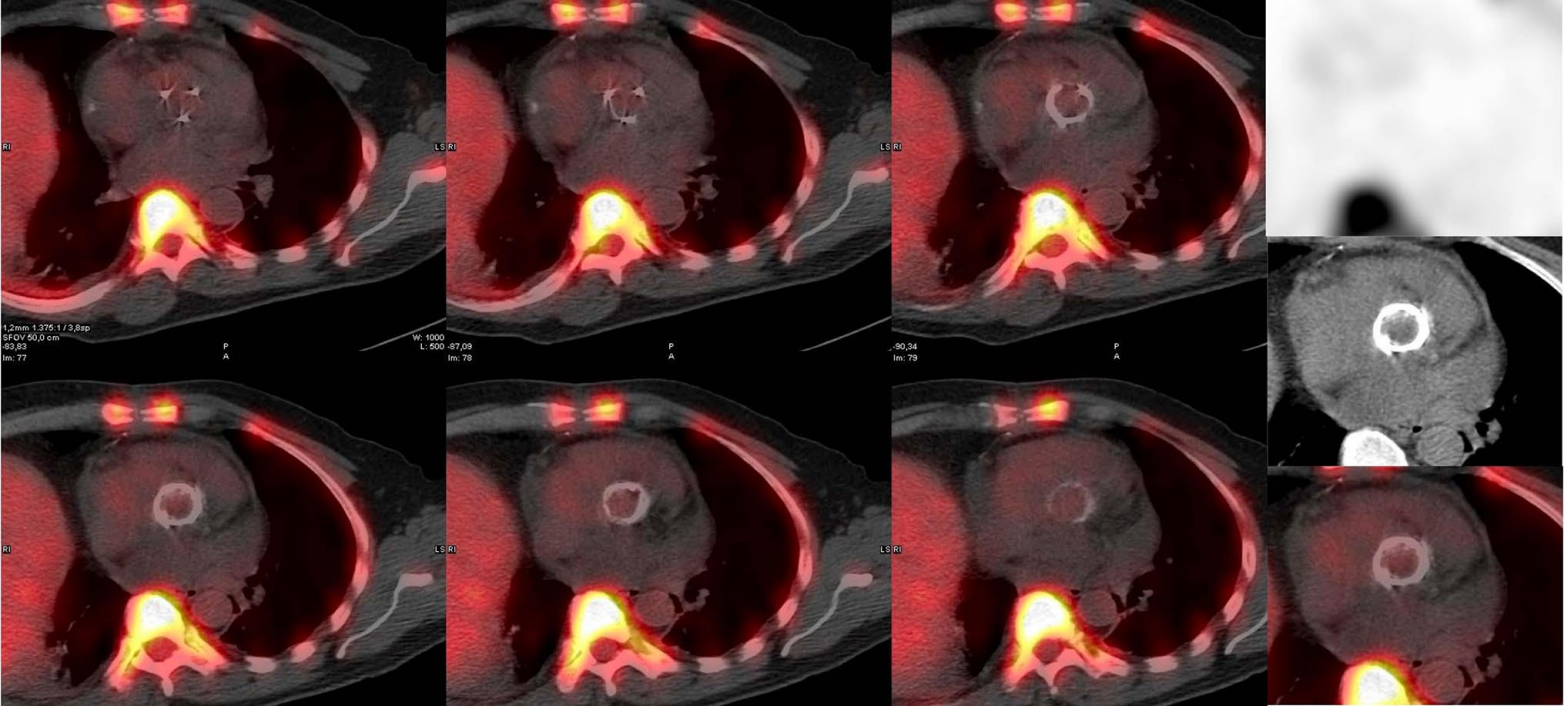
Example of a normal pattern of WBC uptake (^99m^Tc-HMPAO WBC) in a non-infected aortic valve as shown by the re-oriented SPECT/CT images acquired 24 h after the radiopharmaceutical injection (left panel superimposed SPET/CT and right panel top-down emission, CT, and superimposed SPECT/CT respectively) on Discovery-670, GE Healthcare
